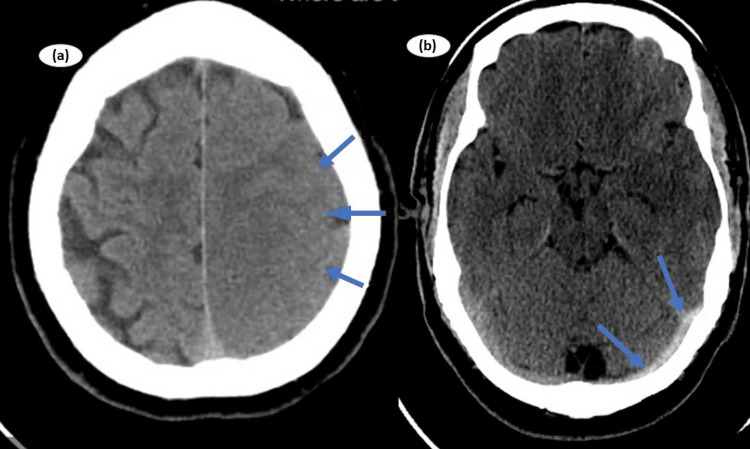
Computed tomography showing vasogenic edema of left cerebral hemisphere (a) and hyperdense left transverse sinus (b)
